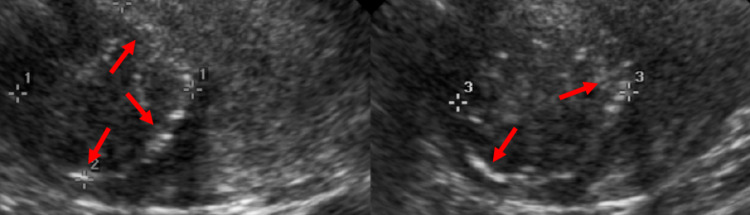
Pelvic ultrasound of the patient’s irregularly shaped uterus, showing a calcified fibroid (red arrows) measuring 5.5 x 5.6 x 5.4 cm.
